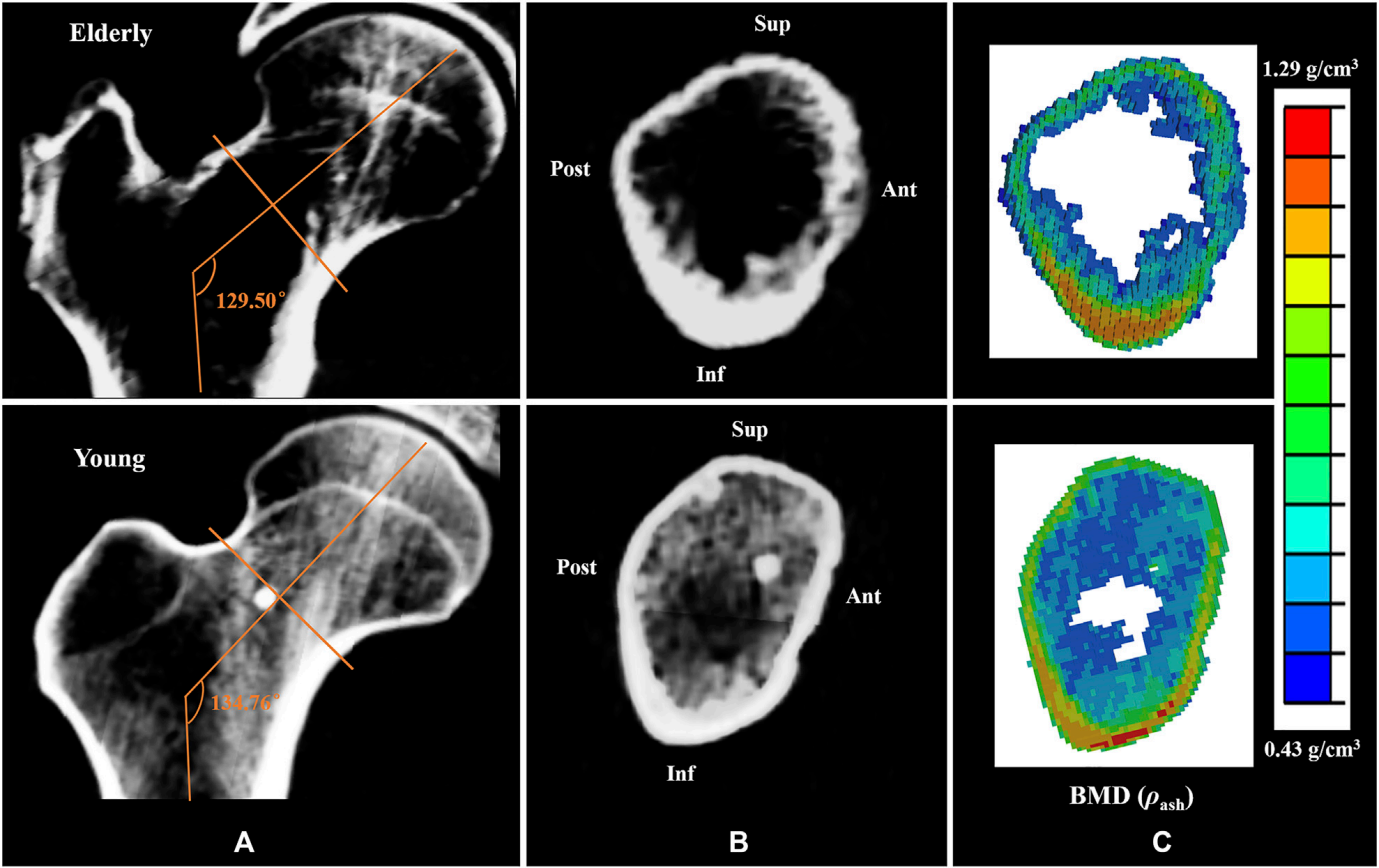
Comparisons of the CT scan slices and the BMD distributions between the elderly and the young. (A,B) the CT scan slices of the proximal femur and the mid-femoral neck, (C) the BMD distributions of the mid-femoral neck.
